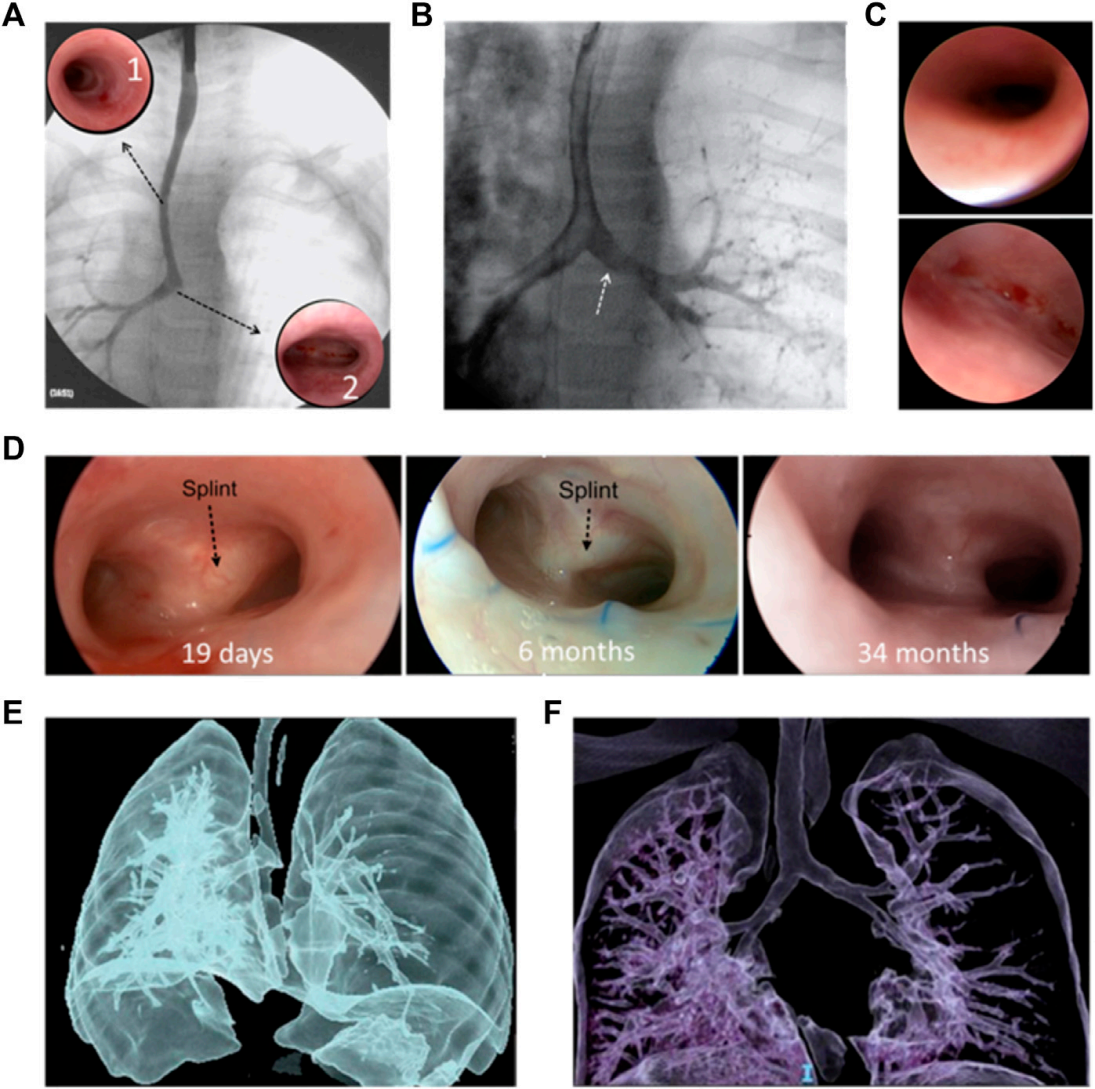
Clinical evaluation of a patient with BM pre- and post-operative: Pre-operative: (A) tracheobronchography with long tracheal stenosis, good caliper right bronchus, and absence of contrast passage to the left bronchus. Complete stenotic tracheal rings (A1); normal carina and origin of the right bronchus (A2). (B) With pressure inside the airway, passage to the left bronchus (white arrow). (C) right bronchus of normal diameter (top) and Malacic left bronchus (bottom), without a lumen. (D) Post-operative: Good bronchial lumen 19 days (left) and 6 months (middle) after surgery; black arrows indicate the splint observed through the mucosa. 34 months after the operation no splint was observed (right) and the airway lumen remains stable. CT: pre-operation (E) and 18 months after surgery (F) where adequate tracheal and bronchial lumen are observed.
